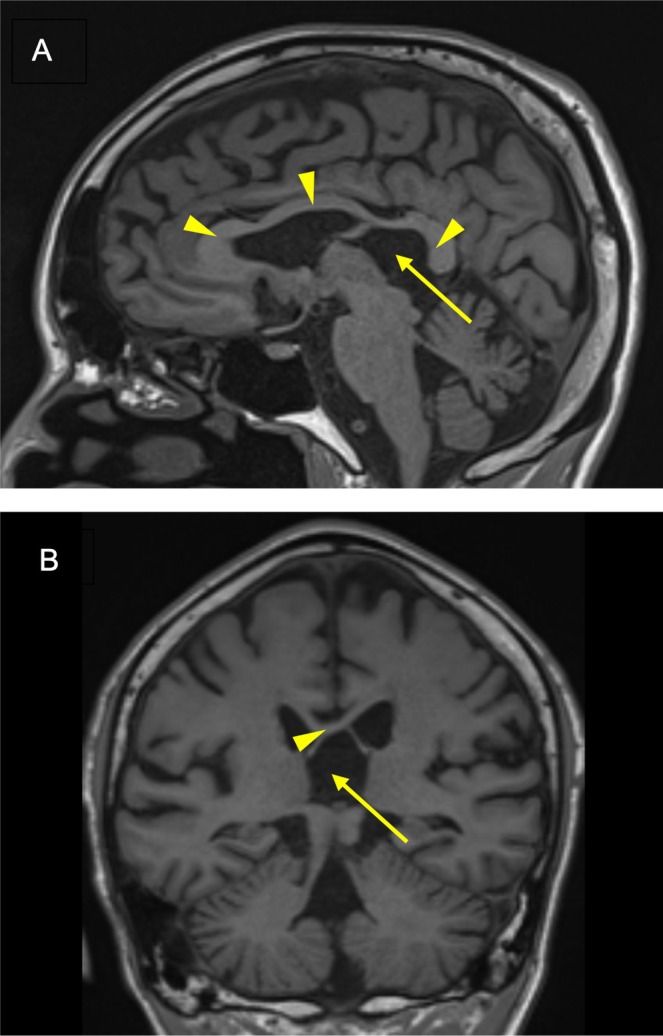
MR scan of brain. (A) Sagittal T2‐FLAIR‐weighted image; (B) Coronal T2‐FLAIR‐weighted image demonstrating dysgenesis of the corpus callosum (yellow arrowheads) and cavum veli interpositi (yellow arrow).
